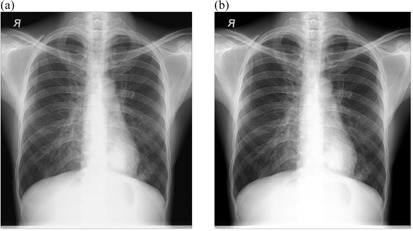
Low kVp chest radiograph: (a) original image and (b) processed image by background subtraction and pixel adjustment.
